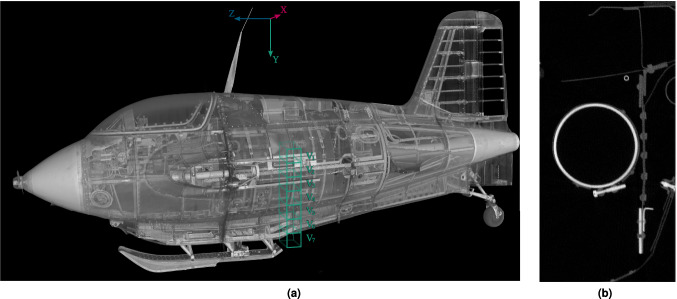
Rendering of the reconstructed fuselage of the scanned airplane (a) and detail (b) located at approximately the midpoint of the fuselage between the nose and the tail of the airplane. The green () boxes in b mark the positions of the extracted sub-volumes. The same Figure also shows the coordinate axis of the CT scan of the front part of the fuselage used for the sub-volumes.
